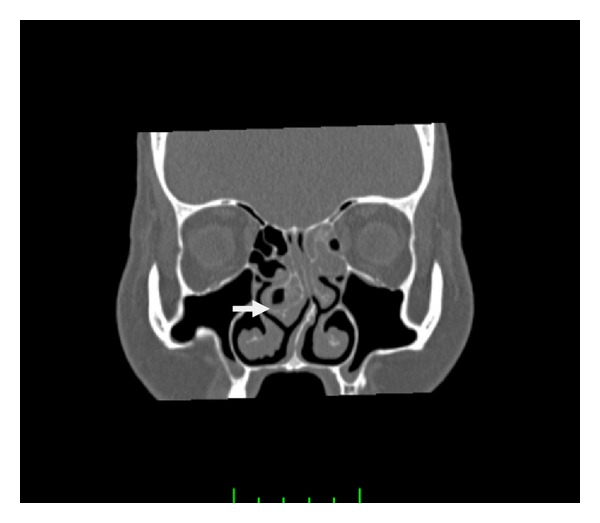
Coronal section of paranasal sinus on a noncontrast CT scan, showing partial heterogeneous opacification of the right concha bullosa, within which hyperdense foci are observed.
